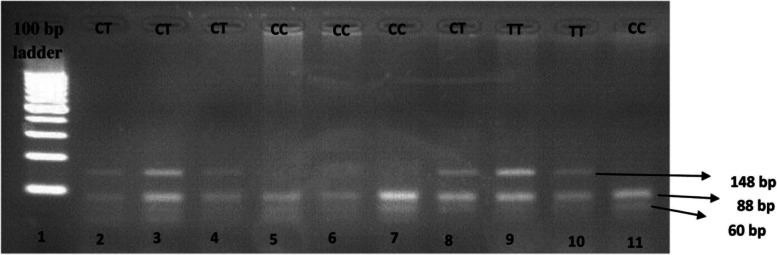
The gel shows all the possible genotypes. The samples (50 ng) were loaded and ran against a 100-bp DNA ladder marker. In the case of homozygous wild CC as in lanes 5, 6, 7, and 11, 3 bands were obtained (88, 82, and 60bps). In the case of heterozygous CT as in lanes 2, 3, 4, and 8, 4 bands were obtained (148, 88, 82, and 60bps), while for homozygous minor TT as in lanes 9 and 10, only 2 bands were obtained (148 and 82bps)
